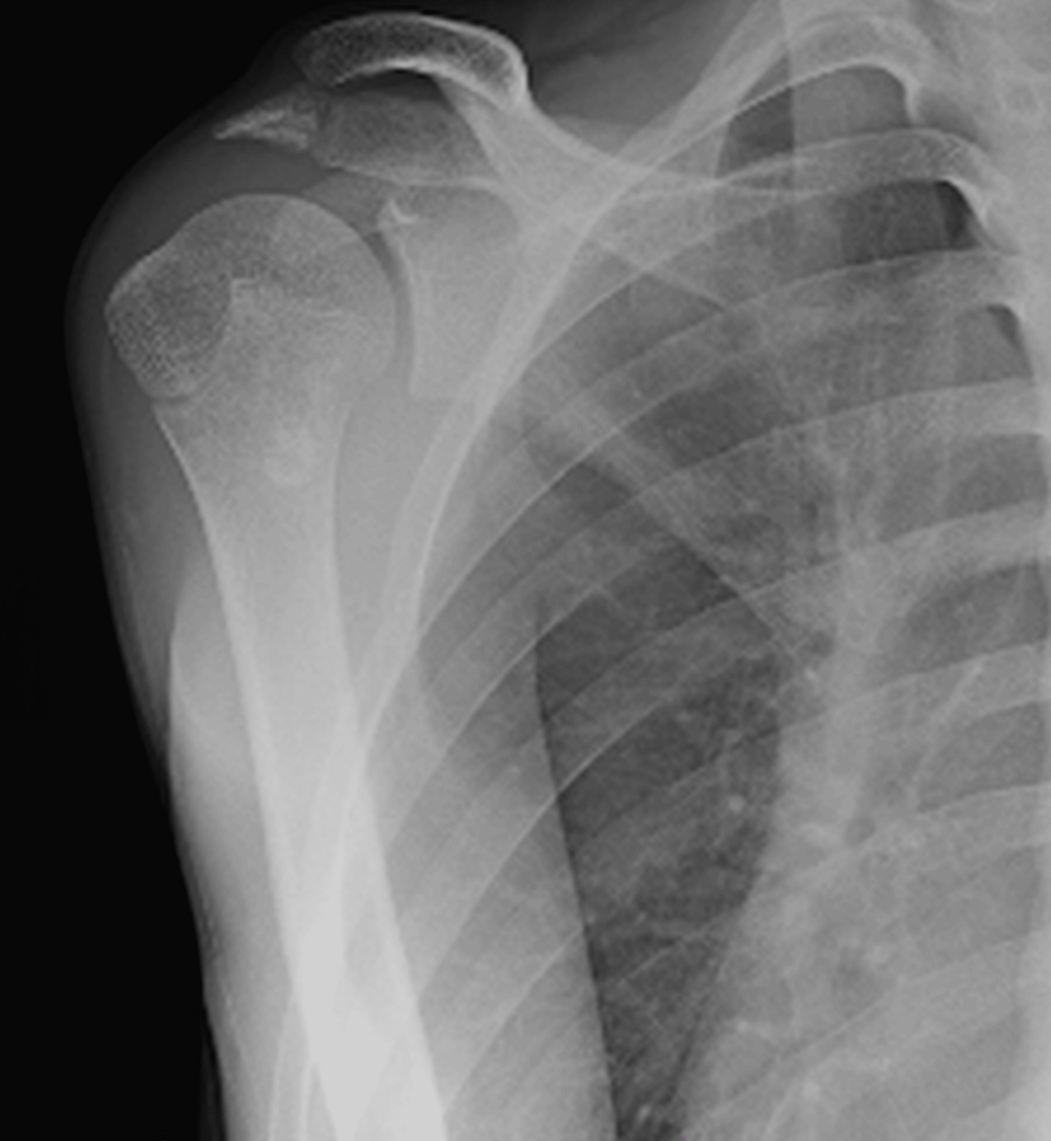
Plain radiography on the frontal view
